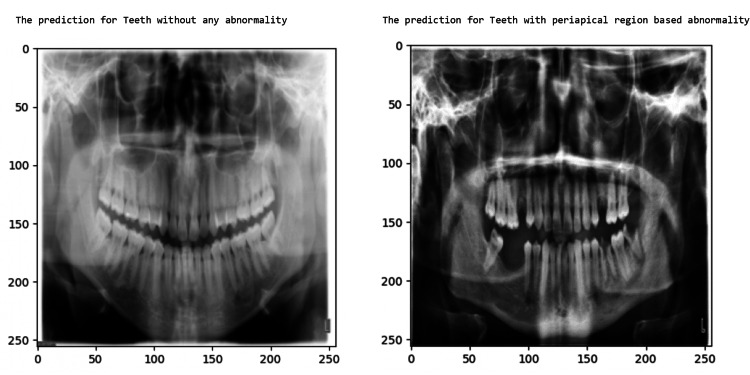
Dental radiograph with periapical region-based abnormality predicted by the model
